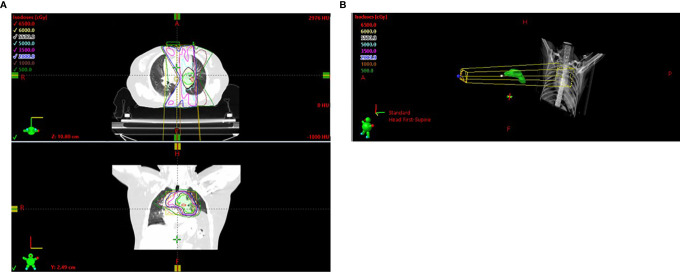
(A) Radiation field. (B) DRR in the AP. DRR, digitally reconstructed radiograph; AP, anterior–posterior.
